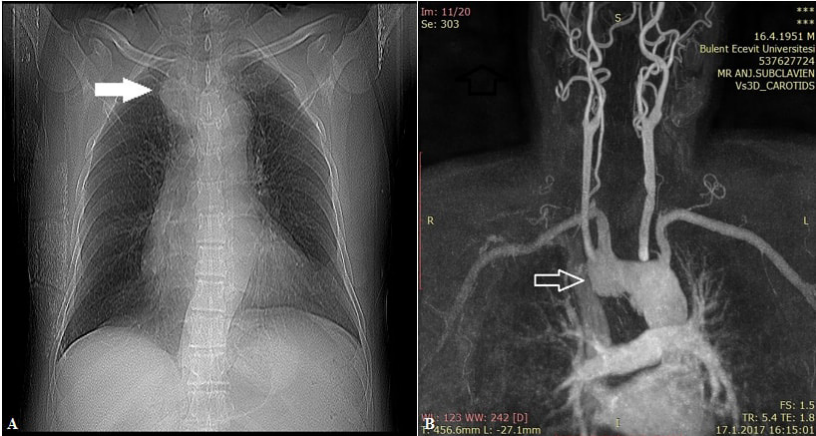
Chest radiogram and MRI of the patient.
